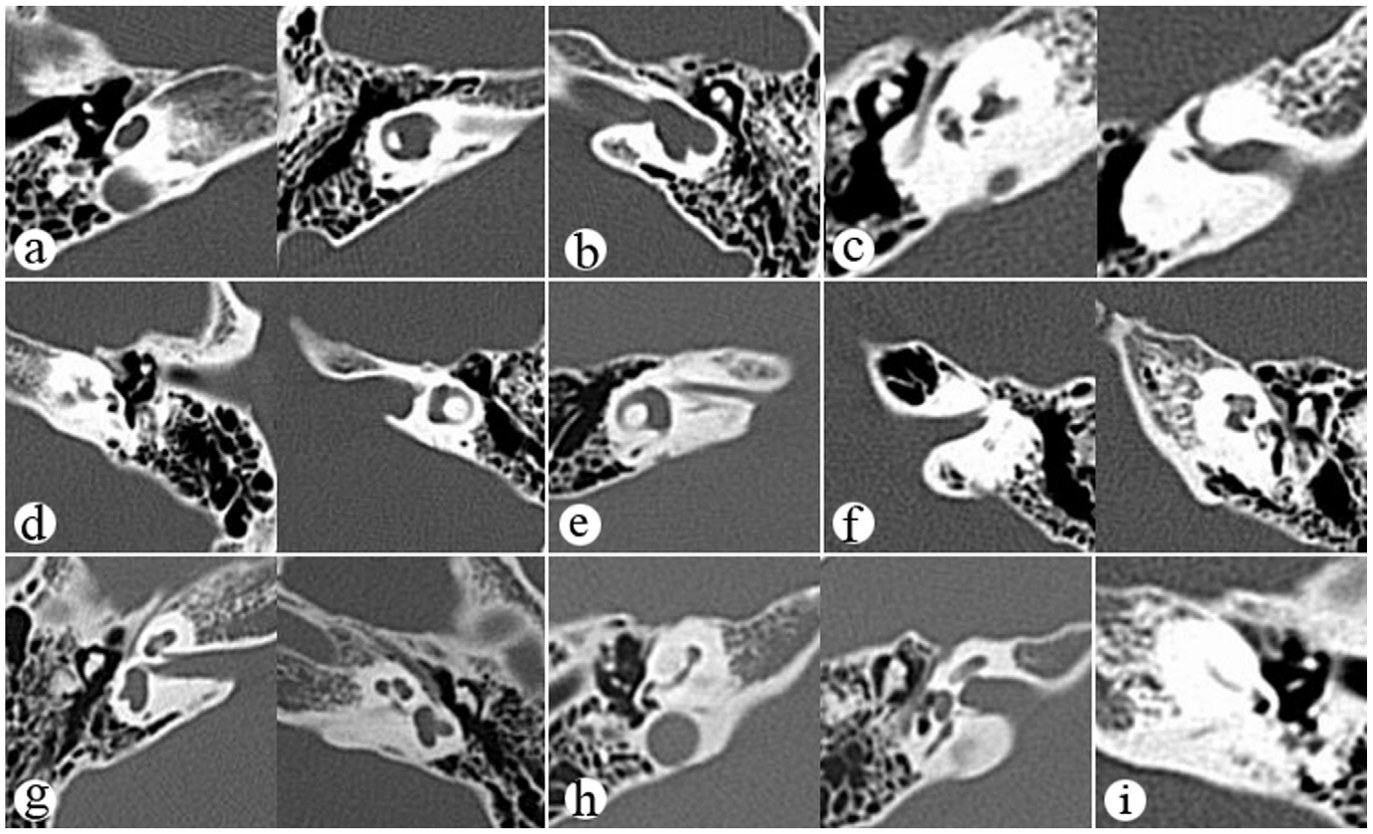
CT images of inner ear malformation in Tibetan patients with hearing impairment. a. incomplete partition type I b. common cavity deforrmity c. malformation of inner ears including cochlea,vestibular and semicircular canals d. malformation of cochlea and enlargement of internal auditory canals e. narrow internal auditory canal f. malformation of vestibular and semicircular canals g. malformation of semicircular canals h. malformation of cochlea i. ossification of the inner ear.
